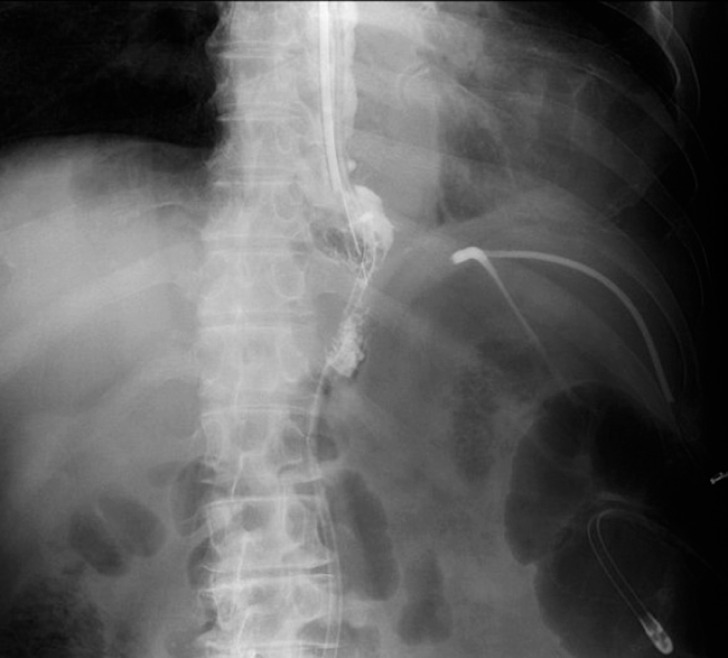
Radiographic image showing resolution of the suture failure.
